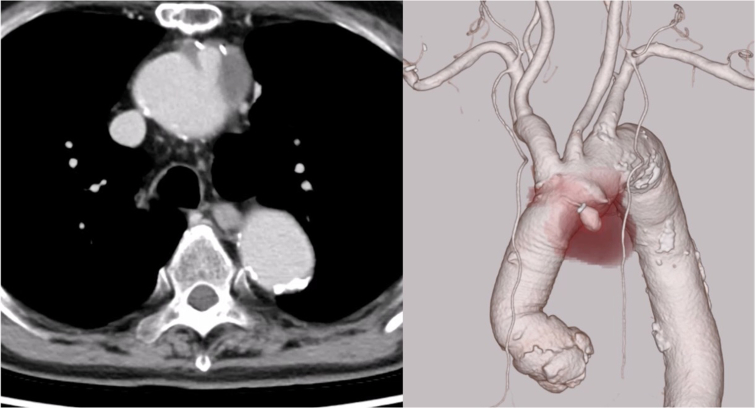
Follow-up computed tomography scan performed 6 years after ascending aortic replacement with a 26-mm J Graft Shield Neo showing a pseudoaneurysm at the anastomotic site on the lesser curvature of the aortic arch, gradually enlarging to 38 mm.
